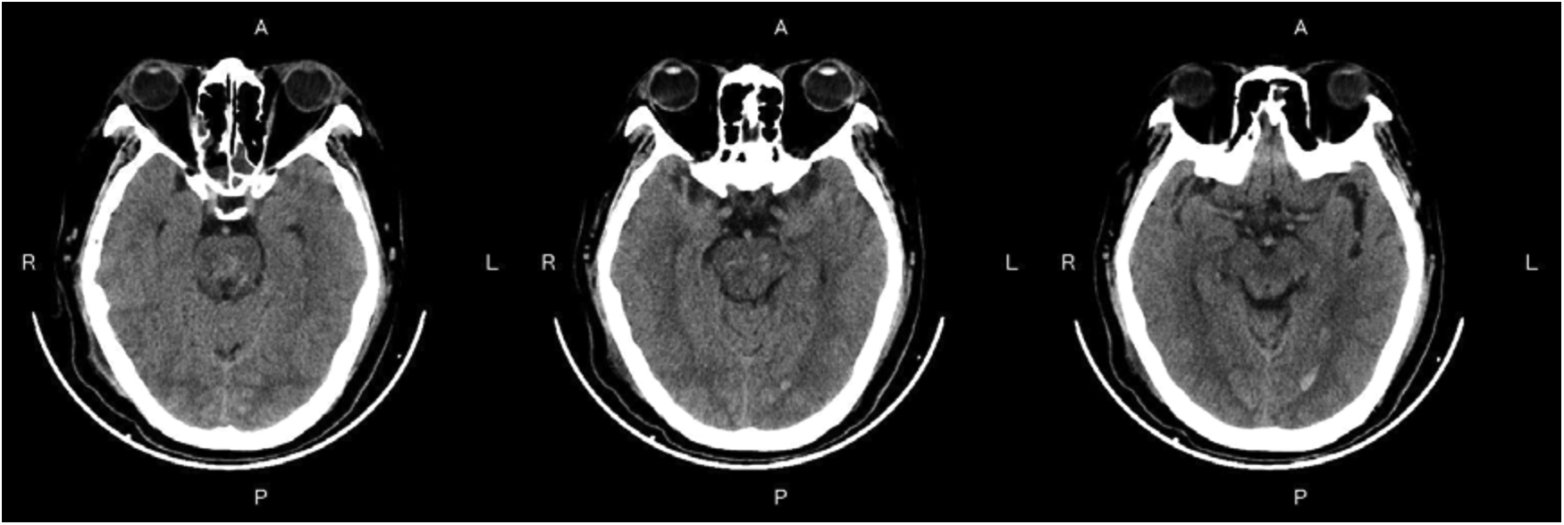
Postoperative imaging: Figure 5 presents a postoperative CT scan of the same patient, obtained 15 days after the stereotactic aspiration procedure.
